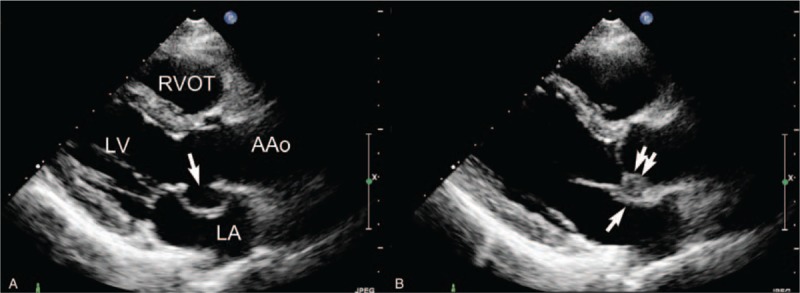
Two-dimensional echocardiography demonstrating P-MAIVF. (A) Left ventricular long-axis view shows a pocket-like echo-free lesion protruding into the left atrium in the mitral-aortic intervalvular fibrosa region during systole. The lesion involved the wall of the noncoronary sinus of Valsalva (single arrow). (B) The lesion collapses during diastole (single arrow); the aortic valve prolapse and vegetation are also observed (double arrow). AAo = ascending aorta; LA = left atrium; LV = left ventricle; P-MAIVF = pseudoaneurysm of the mitral-aortic intervalvular fibrosa; RVOT = right ventricular outflow tract.
